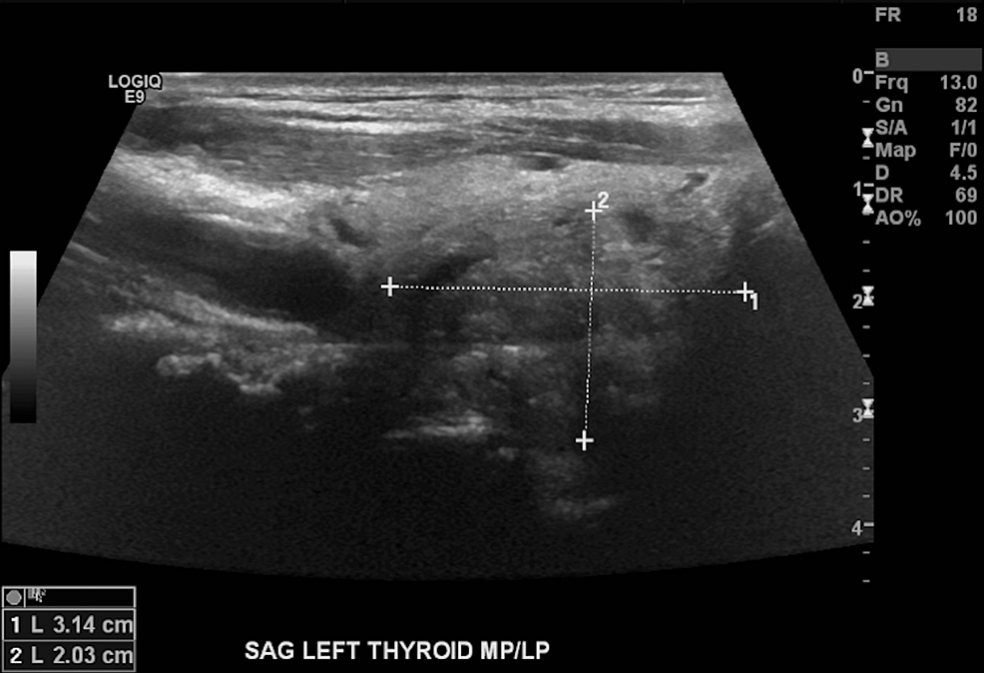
Thyroid ultrasound after treatment with methimazole showing a nodule in the mid to lower pole on the left, measuring 3.1 x 2 x 2 cm
